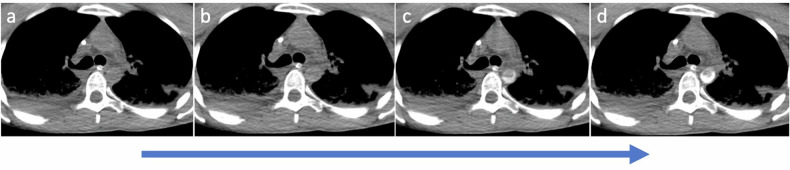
Computed tomography (CT) bolus tracking at the level of the distal ascending aorta in a femoro-femoral veno-arterial (VA)-extracorporeal membrane oxygenation (ECMO) supported patient showing retrograde contrast flow with the enhancement of the descending aorta before the ascending aorta. Contrast-blood layering is visible in the descending aorta (c, d), whereas no enhancement of the ascending aorta is identified in the earlier phases (a, b)
